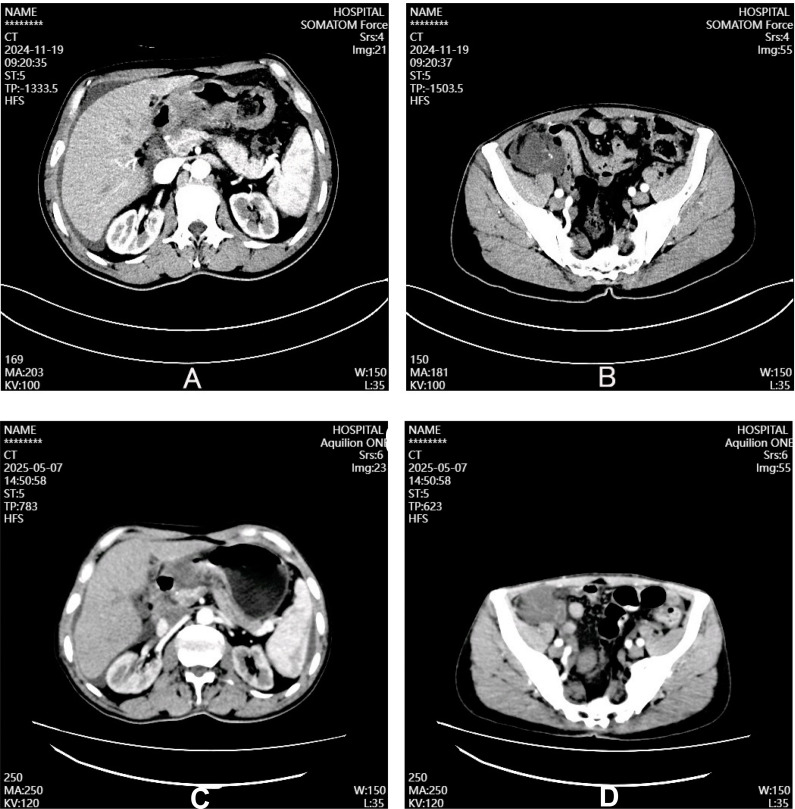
Contrast-enhanced CT findings before (A, B) and after (C, D) neoadjuvant chemoimmunotherapy. Baseline imaging demonstrated marked gastric wall thickening with luminal narrowing (A), omental infiltration, moderate ascites, and a cystic lesion with irregular wall thickening in the right iliac fossa (B). Following six cycles of XELOX plus sintilimab, significant regression of gastric wall thickening was observed with improved luminal patency (C); however, ascites persisted and the right lower quadrant cystic lesion remained essentially unchanged (D).
